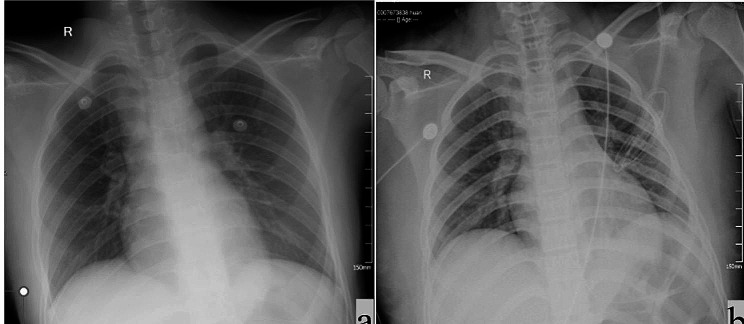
Chest orthographic radiography results: a Case A on the first day of admission and b Case B on day 5 of admission, both revealing no obvious abnormalities
